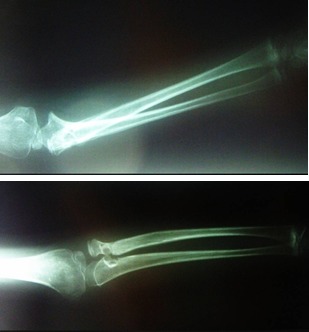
Case 1: X-rays two months after surgery. Images reflect normal position of radial head relative to the ulna and the humeral condyle
